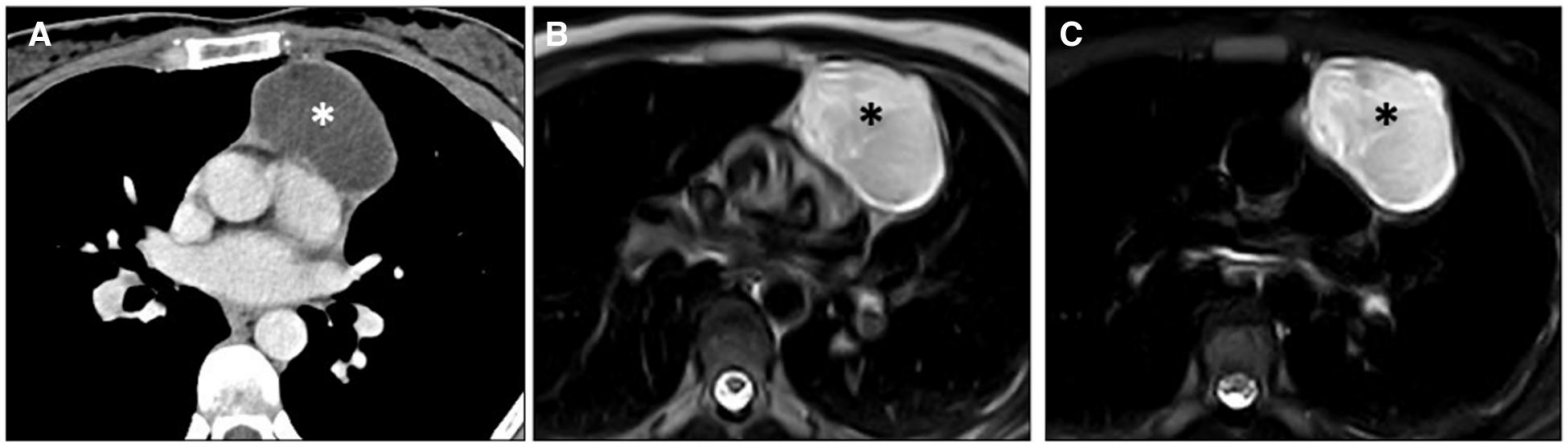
Thymic cyst. (A) Contrast-enhanced axial CT image of the chest shows a prevascular uniloculated thin-walled lesion (white asterisk) with fluid attenuation, in the typical location of the thymic structure. (B) Axial T2-weighted MR image shows hyperintense signal intensity of the lesion (black asterisk), maintained in fat-suppressed sequence (C) and confirming its water content.
